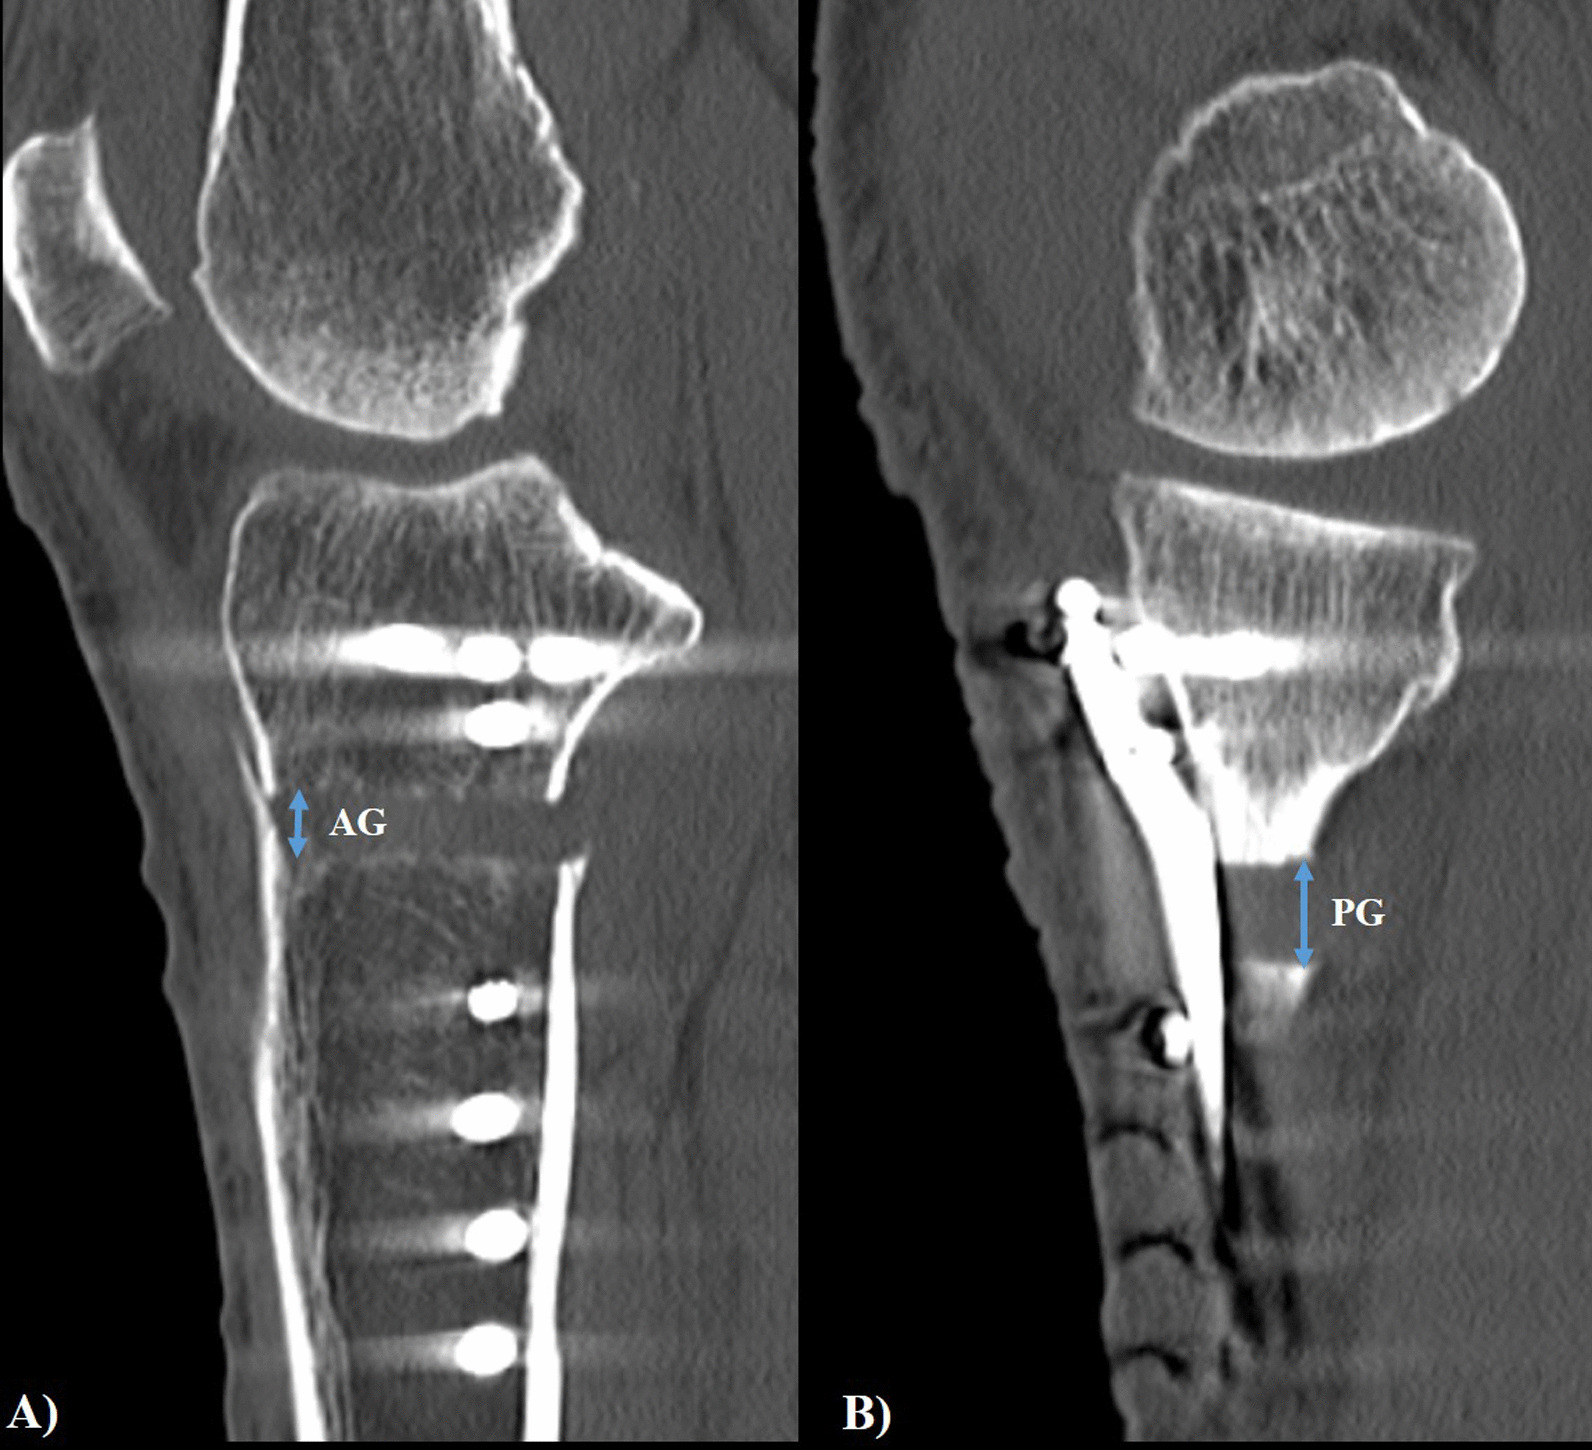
Gap measured on computed tomography (CT). a The anterior gap is measured at the most medial part of the tibial tubercle and b the posterior gap is measured at the most medial and posterior height of the osteotomy site using the CT sagittal view
